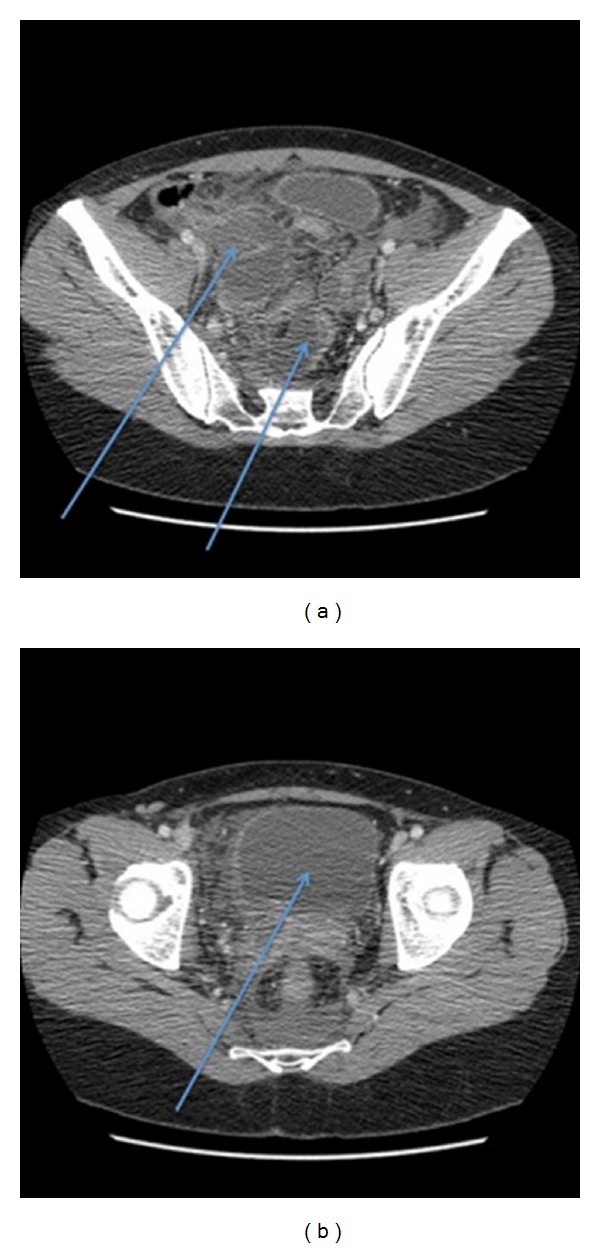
CT scan of the abdomen and pelvis, with contrast agent injection, on postoperative day 8, no excretory phase images. No urologic lesions were detected. (a) Presence of 2 pelvic collections (45 and 35 mm) and (b) full bladder.
